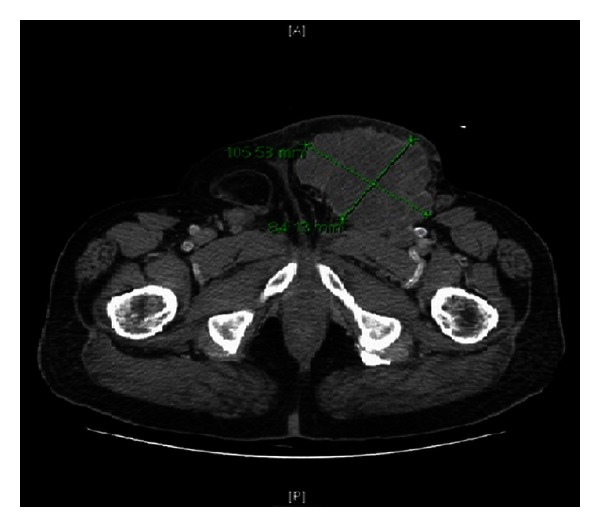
Computed tomography (CT) scan with contrast showing an 8.5 × 10.5 cm, heterogeneous, lobulated, and large mass in the left groin, compressing the left common femoral vein and inseparable from the vein as well as from the adductor muscles ventrally. The mass is associated with local lymphadenopathy, multiple small subcutaneous nodules, and an enlarged left external iliac lymph node.
